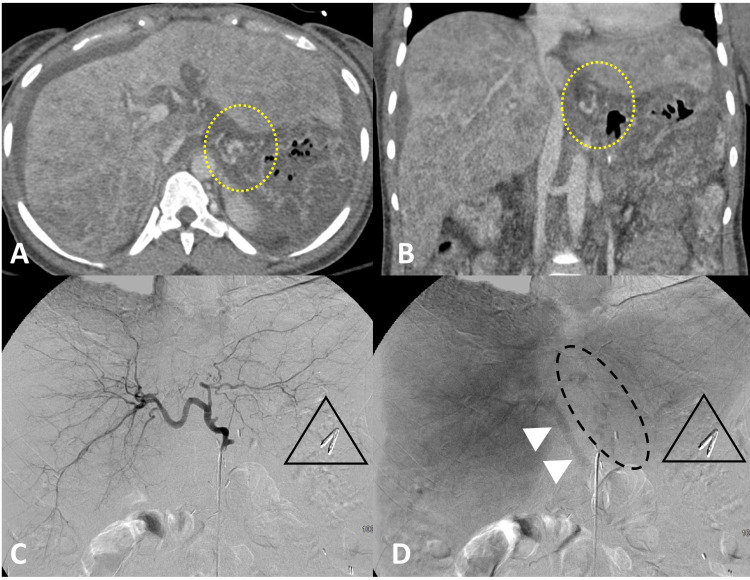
CT imaging of the abdomen and hepatic angiogram Contrast-enhanced CT imaging of the abdomen in the delayed, portal venous phase, 100-second delay, (A and B) demonstrates a nodular/lobulated liver contour with increased heterogeneity throughout the hepatic parenchyma with evidence of sequela of portal hypertension, including gastroesophageal varices (yellow perforated circle). Axial slice (A). Coronal slice (B). Hepatic angiogram images (C and D). Early arterial phase (C) showing no evidence of active arterial bleeding, as well as the presence of endoscopy clips (black triangle). Delayed portal venous phase images from the hepatic angiogram (D) demonstrate patency of the portal vein (white arrowheads) and faintly opacified esophagogastric varices (perforated black oval), as well as presence of endoscopy clips (black triangle). CT: computed tomography.
